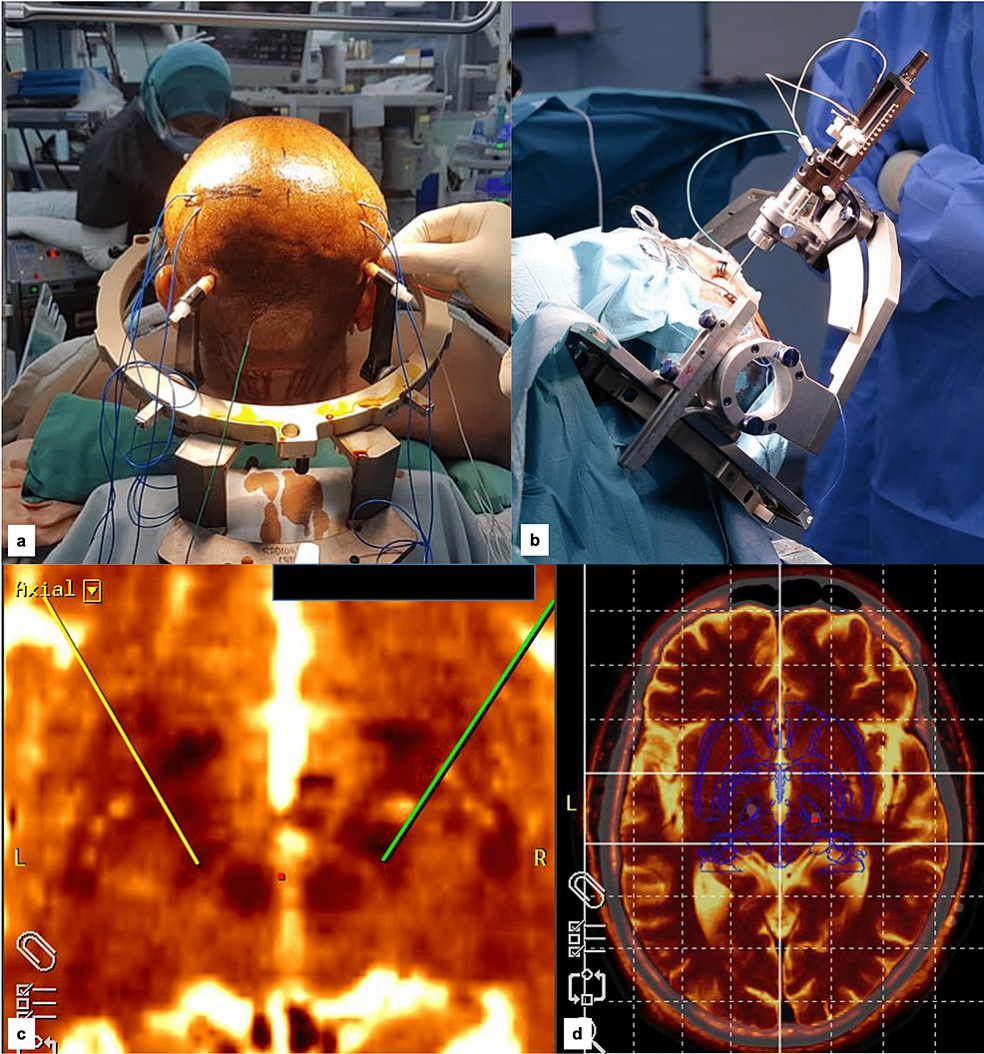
STN-DBS procedure and hand sensorimotor needle scalp EEG (a) Area of the sensorimotor cortex was identified topographically and needle EEGs were inserted deep into the pericranium. (b) STN-DBS procedure using Cosman–Roberts–Wells (CRW) head frame. (c) Bilateral STN targeting and planning based on Framelink. (d) The permanent electrode final site was confirmed with image fusion of the postoperative CT axial image with the preoperative MRI (white and red dot). STN: subthalamic nucleus; DBS: deep brain stimulation; EEG: electroencephalogram
